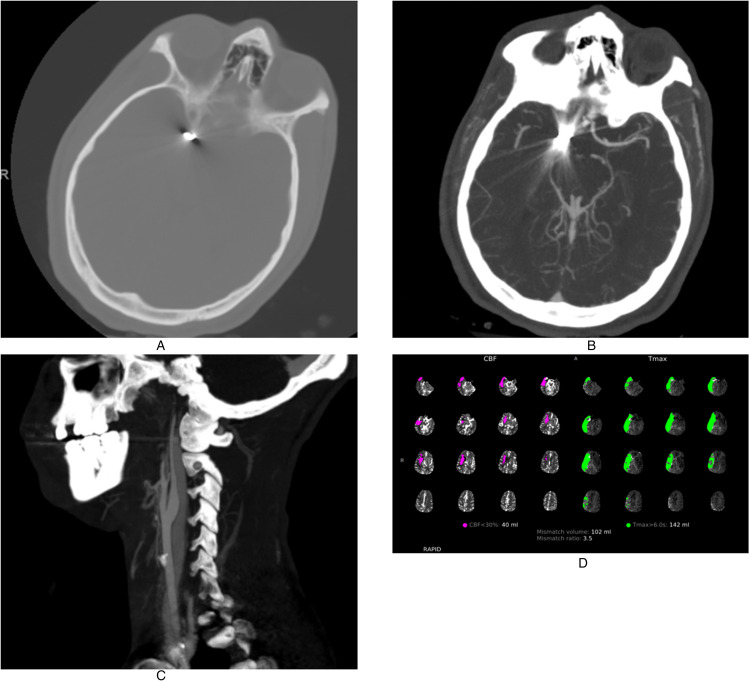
(A) CT head demonstrating metallic fragment with intact calvarium. (B) CTA head showing filling defect in right MCA. (C) CTA neck demonstrating ICA vasospasm starting at the level of C3. (D) Perfusion scan showing large mismatch between penumbra and core infarct.
